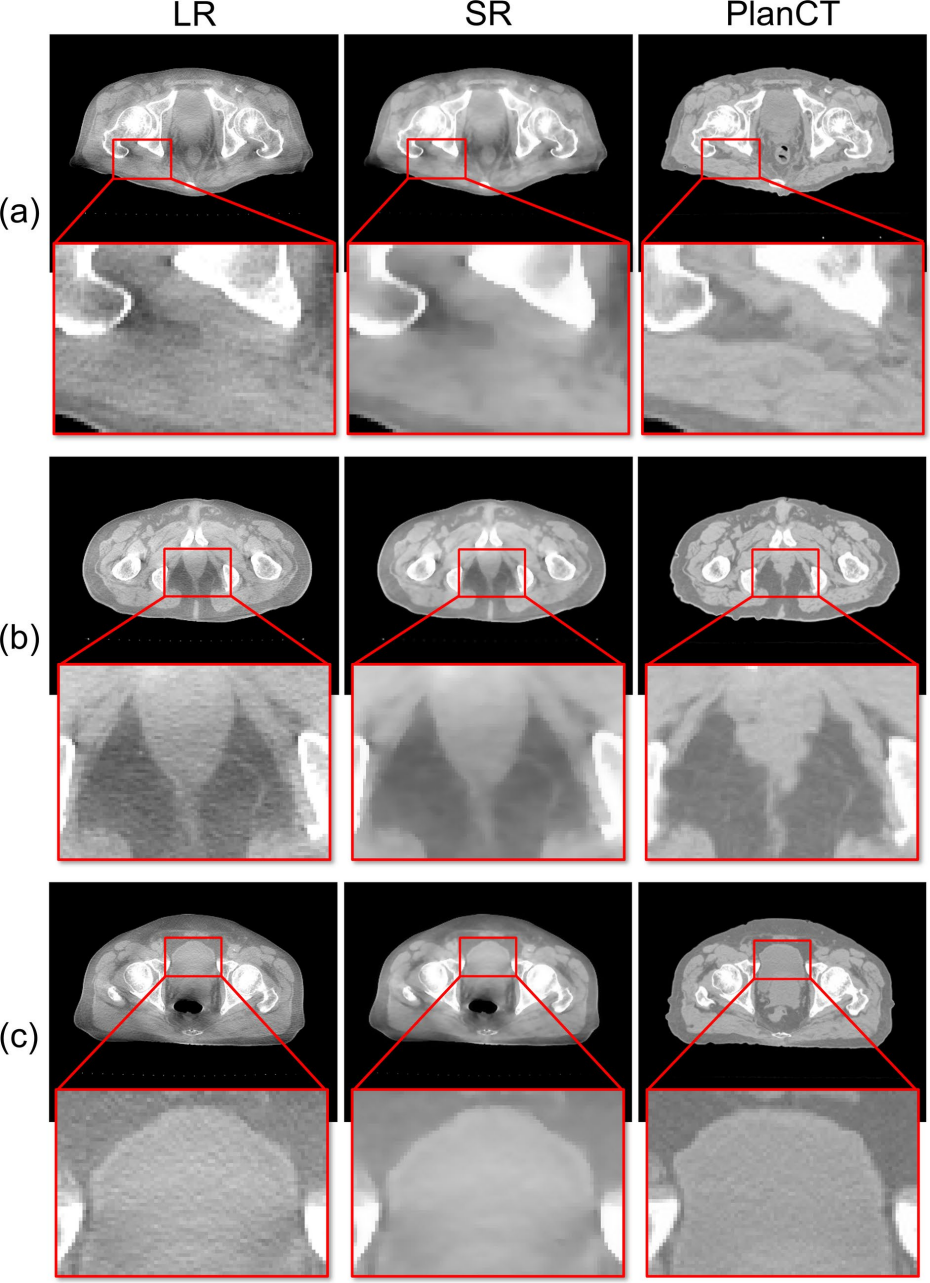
Exemplary images of application to pelvis CT images. CBCT images before processing (LR column). Processed image-quality improvement images (SR column). Treatment planning images with the same slice as the CBCT image (PlanCT column). a, b, and c, respectively, show images of different patients, depicting enlarged images of muscle and fat (a), rectum (b), and bladder (c). The CT number display window ranges from [− 840, 600] HU
